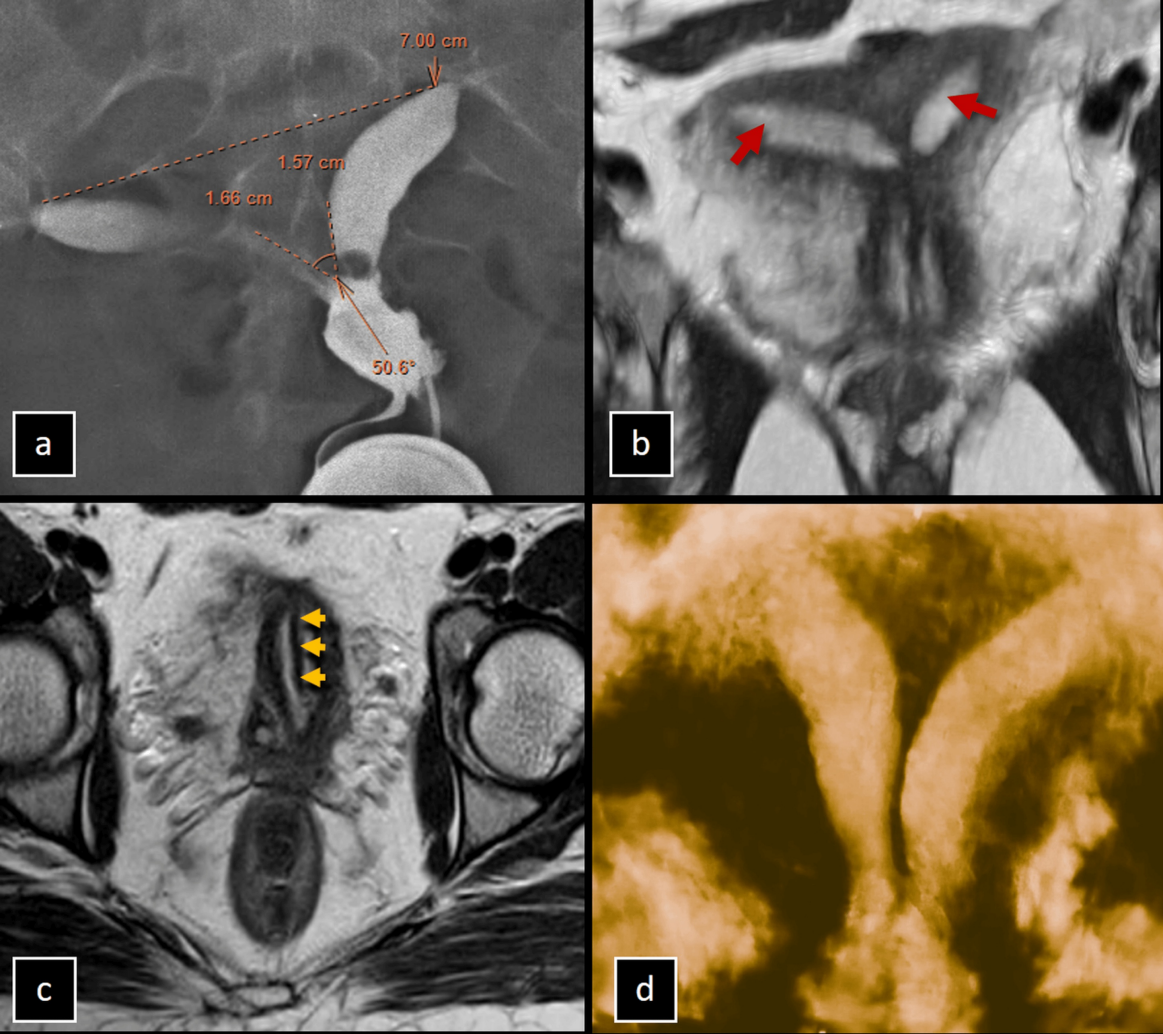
(a-d) Imaging of the Complete Septate Uterus in a Patient with Infertility. (a) Hysterosalpingogram (HSG) image of a 27-year-old female with infertility shows contrast opacification of a single cervical canal with two discrete, spaced uterine cavities, an inter-cornual distance of approximately 7 cm, and an inter-cornual angle of approximately 51 degrees, which is equivocal between bicornuate unicollis and complete septate uterus. (b) MRI oblique axial T2-weighted maximum intensity projection (MIP) and T2-weighted axial (c) images confirm the findings of two uterine cavities in the fundus (red arrows) with an intervening septum extending from the fundus to the cervix/vagina (yellow arrowheads). These features favor a diagnosis of a complete septate uterus. (d) 3D ultrasound provided additional information on the septum's thickness and vascularity.
